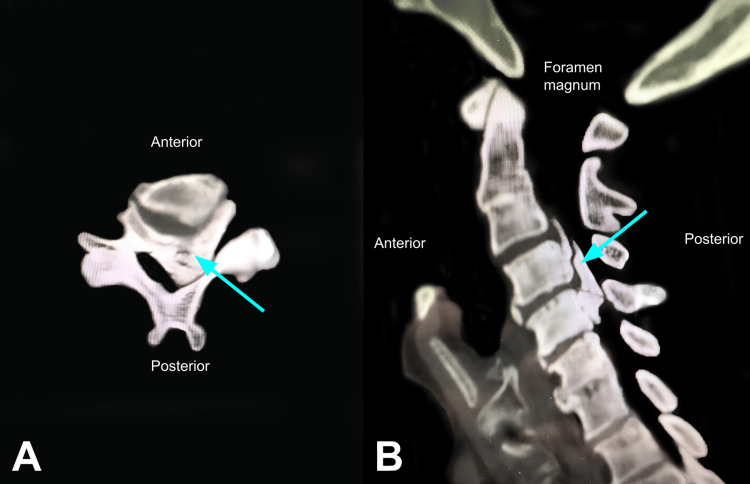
Axial (A) and sagittal (B) views of the cervical CT scan. Ossified posterior longitudinal ligament is seen causing severe stenosis of the spinal canal at the level of C3-C4 vertebral bodies (blue arrow)
